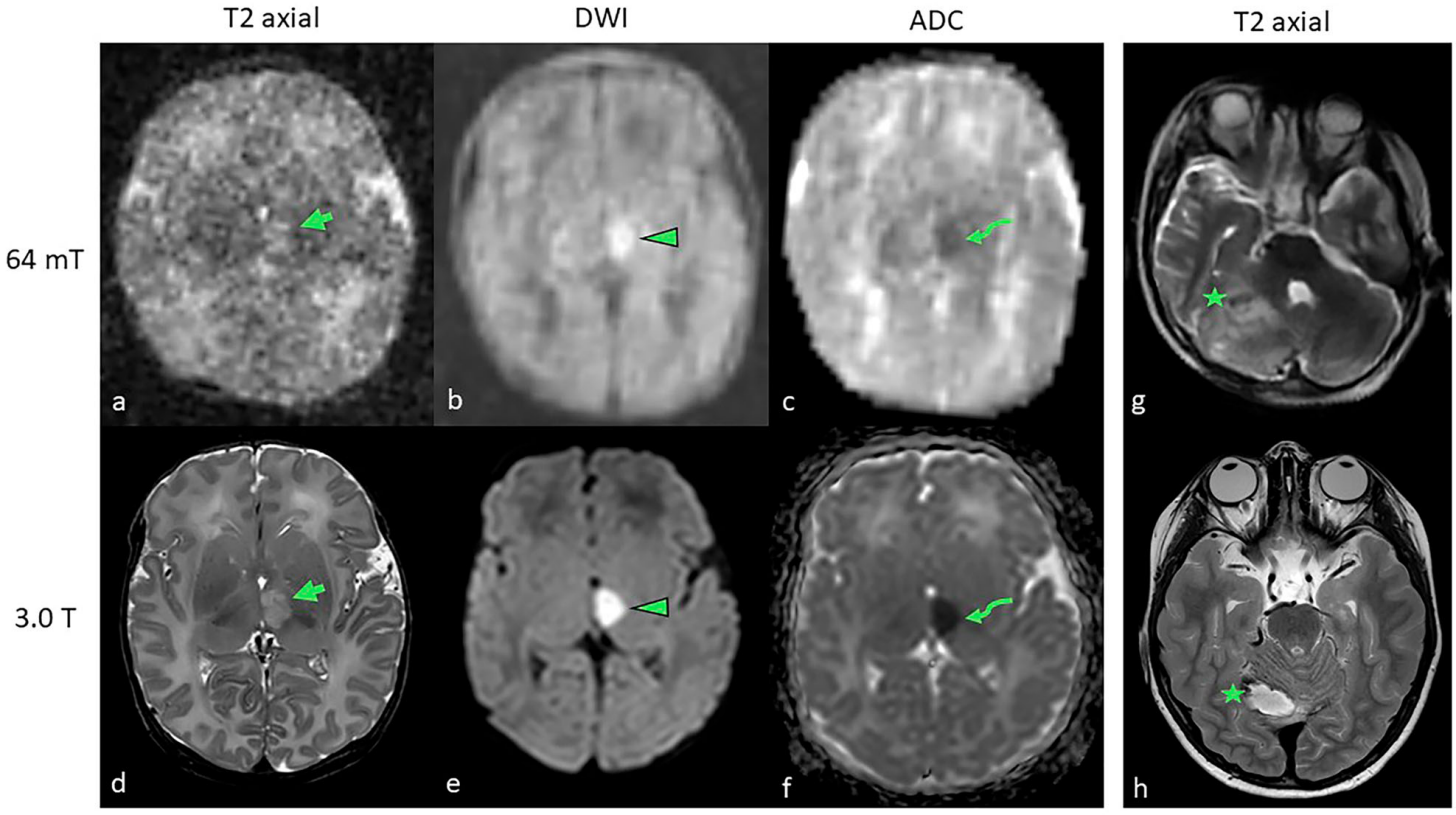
Low-field MRI at 0.064-T (top row) in two different patients (Patient 1, a–f, Patient 2, g, h) compared to 3.0-T (bottom row). Patient 1 (9-month-old male) demonstrates focal T2 hyperintense changes along the left thalamus (arrows) on both low-field (a) and high-field (d) images, as well as on diffusion-weighted images (DWI, b and e, respectively) with correlates on the apparent diffusion coefficient (ADC, c and f, respectively). Patient 2 (5-year-old male) shows a focal T2 hyperintense lesion with mass effect (star) between the brain stem and the right cerebellum as seen on the low-field (g) and confirmed on the high-field (h) sequences. The mass turned out to be an arteriovenous malformation with subarachnoid hemorrhage and edema. The patient required sedation for the high-field scan, while the low-field examination transpired without sedation within 2.2 min in the presence of a parent
